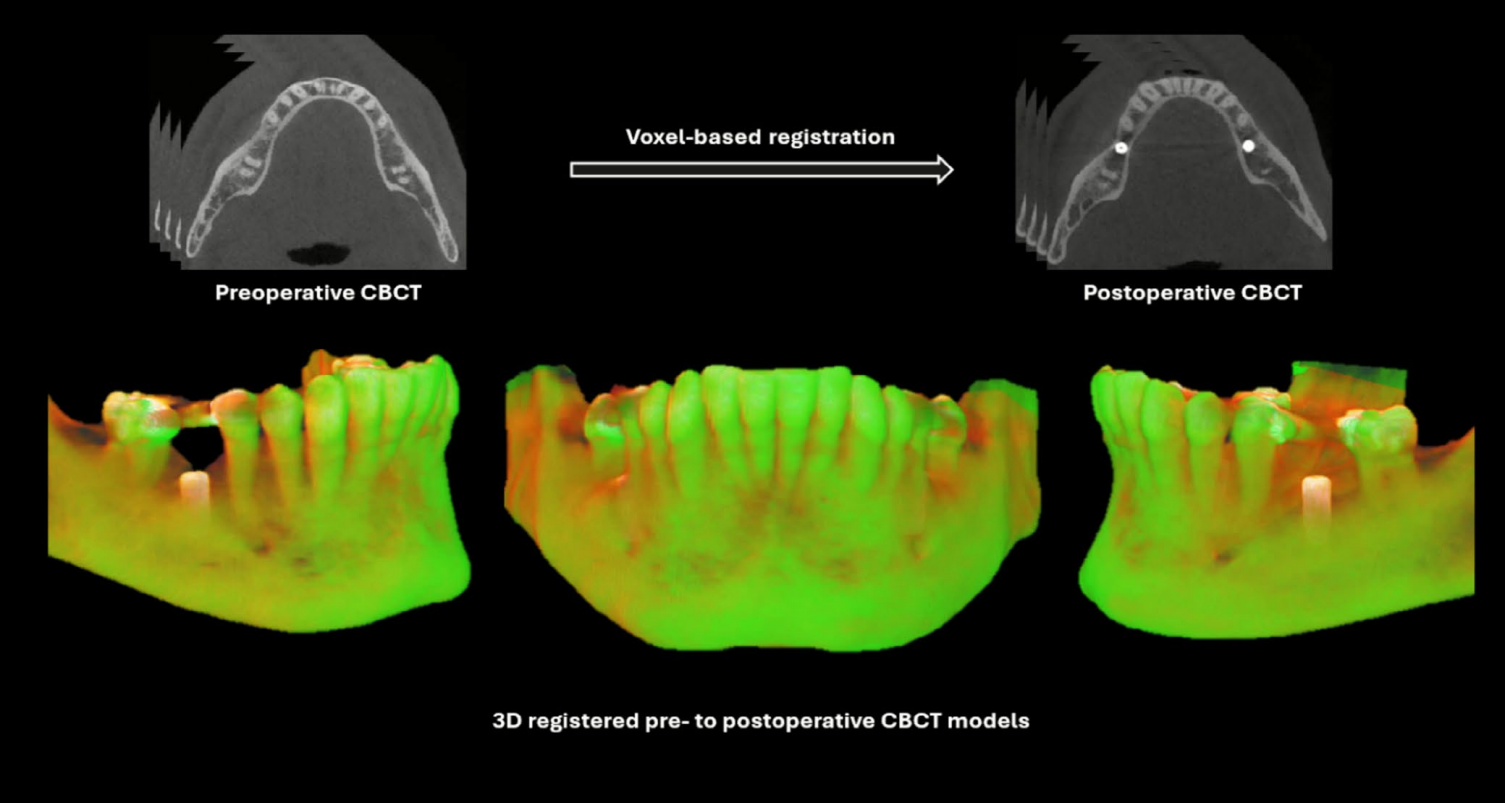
Registration of preoperative and postoperative CBCT scans in Amira software. Green: preoperative CBCT model; yellow: postoperative CBCT model.
